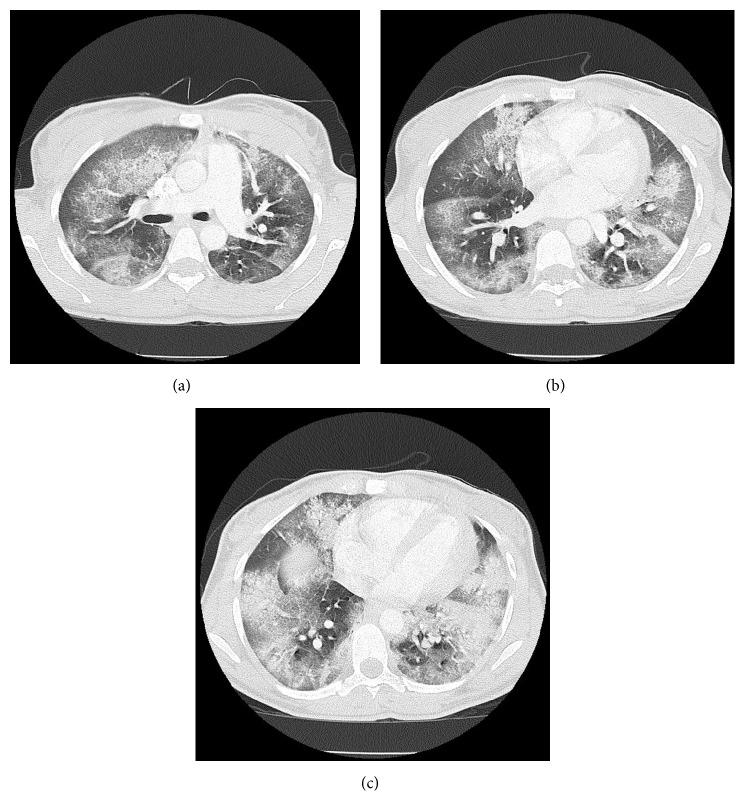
Contrast-enhanced computed tomography demonstrating extensive diffuse ground-glass opacifications, with more confluent opacity involving the lower lobes.
